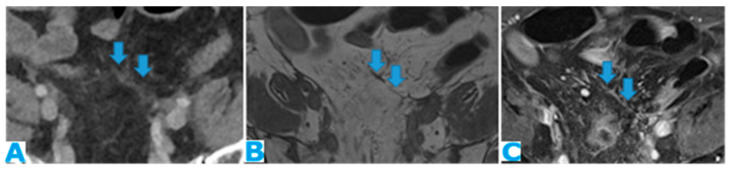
Axial CE-CT (A); axial T2WI (B); axial CE portal phase FS T1WI (C). PC from endometrial carcinoma: deposits seeding within the mesenteric leaves (arrows).
